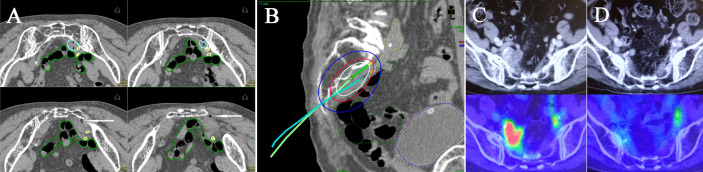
Multi-slice axial CT images (A) of implanted needles and a sagittal CT image (B) of the right internal iliac node (cyan dashed line), bowel (green dashed line), common/internal iliac vessels (yellow dashed line), and bladder (purple dashed line) receiving 50% (blue lines), 100% (red lines), and 150% (white lines) of the prescription dose (6 Gy) in a representative post-operative patient. Pre-treatment CT/^18^F-FDG-PET/CT images (C) and post-treatment CT/^18^F-FDG-PET/CT images (D). Both insertion points and directions were decided using pre-treatment CT. Two flexible interstitial needles (white arrows) were obliquely implanted in the prone position, passing from under the right sacroiliac joint through the front of the piriformis muscle up to the front of the second sacral vertebrae under CT guidance (no contrast medium) in the HDR room with dedicated CT. Implantation was performed under local anesthesia alone, with care taken not to damage the bowel or common/internal iliac vessels. The catheter was inserted into the central part of the node using an iterative approach of catheter implantation and adjustment with serial CT image acquisition. MPR CT was used to reconstruct the axial images into oblique anatomical planes. The patient was irradiated in the prone position in the HDR room with dedicated CT.
